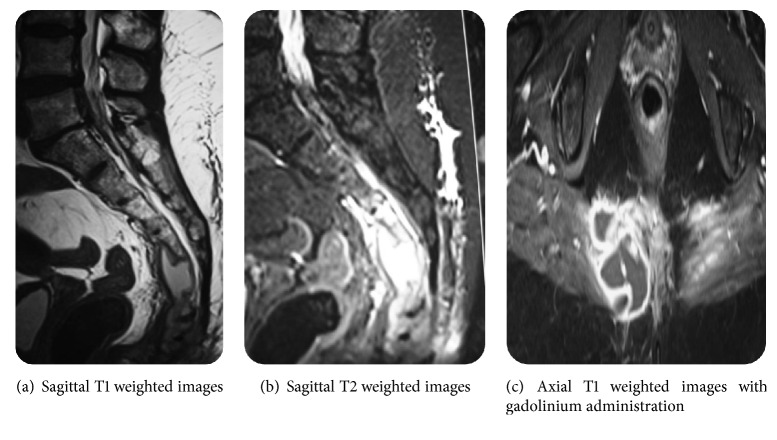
Magnetic resonance images of the patient. Revealing a process of hypointense signals in T1W images (a) and hyperintense signals in T2W images (b), the lesion was moderately enhanced in its periphery after gadolinium administration (c).
